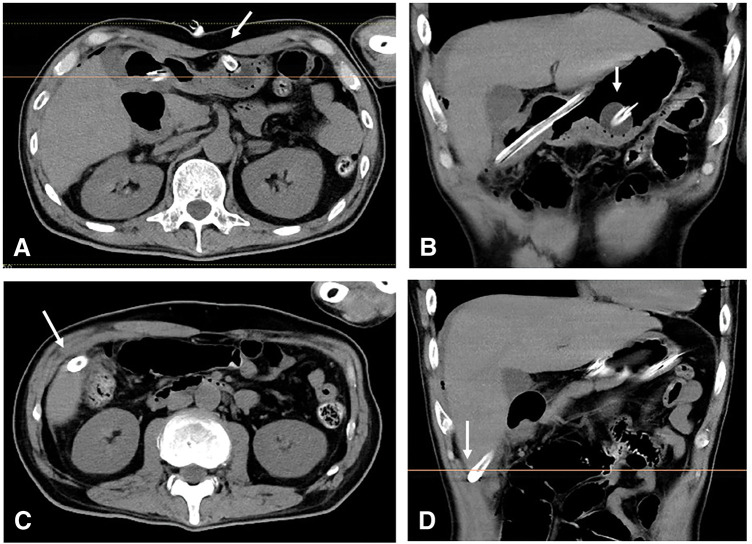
CT performed for acute right-sided abdominal pain on day 12 after PEG-J exchange. (A) Gastrostomy site was fixed at the gastric body (arrow). (B) Gastrojejunostomy tube was secured with a balloon (arrow). (C) Gastrojejunostomy tube was located beneath the liver (arrow). (D) The tube tip was located outside the intestinal lumen (arrow). PEG-J, percutaneous endoscopic gastrojejunostomy
